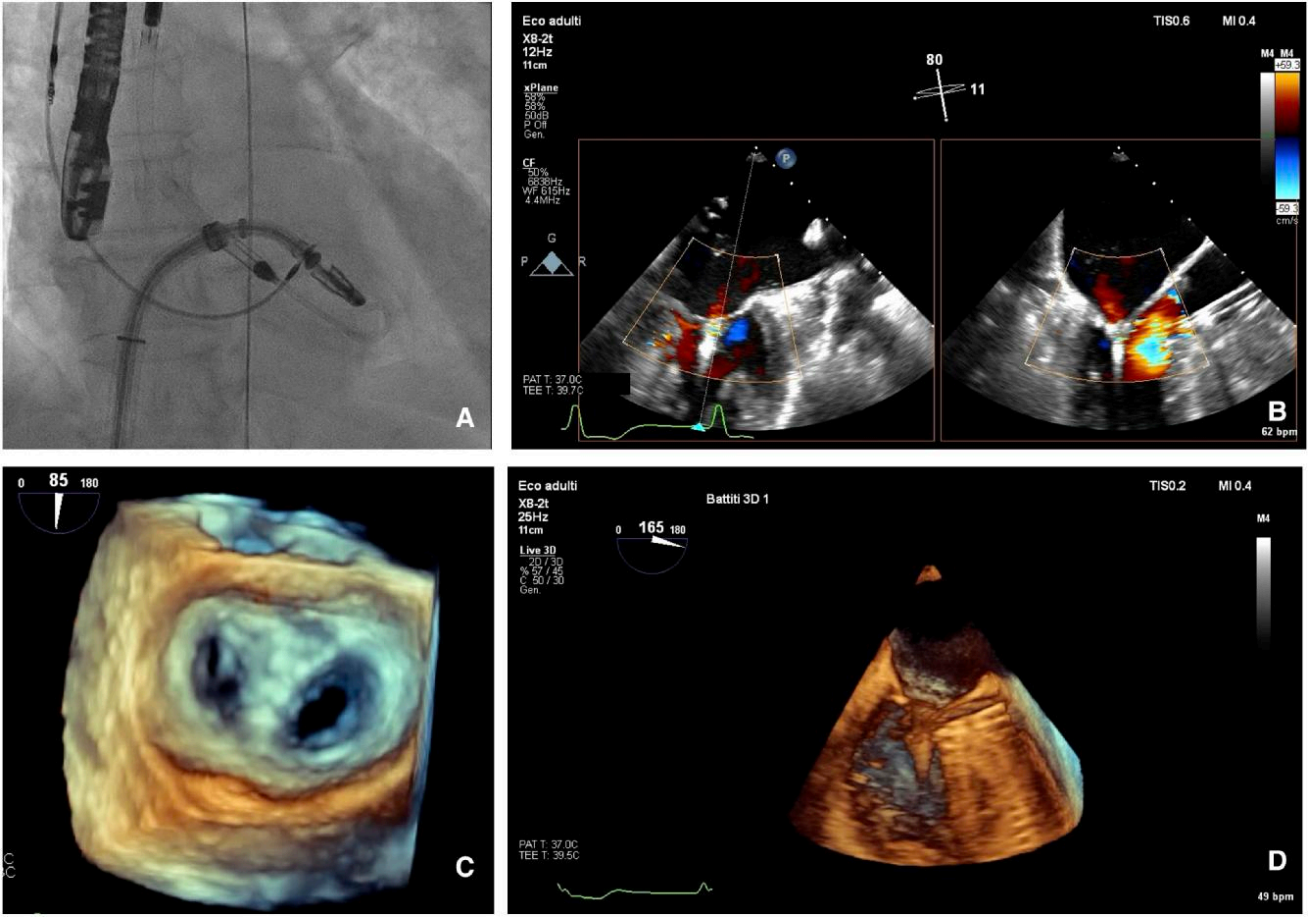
TEER procedure. (*A*) Fluoroscopy showing MitraClip system together with Impella CP in LV. (*B*) TEE showing mild residual MR after MitraClip placement. (*C*) 3D echocardiography of mitral valve after MitraClip implantation. (*D*) 3D echocardiography showing MitraClip and Impella CP catheter in close proximity without interference.
